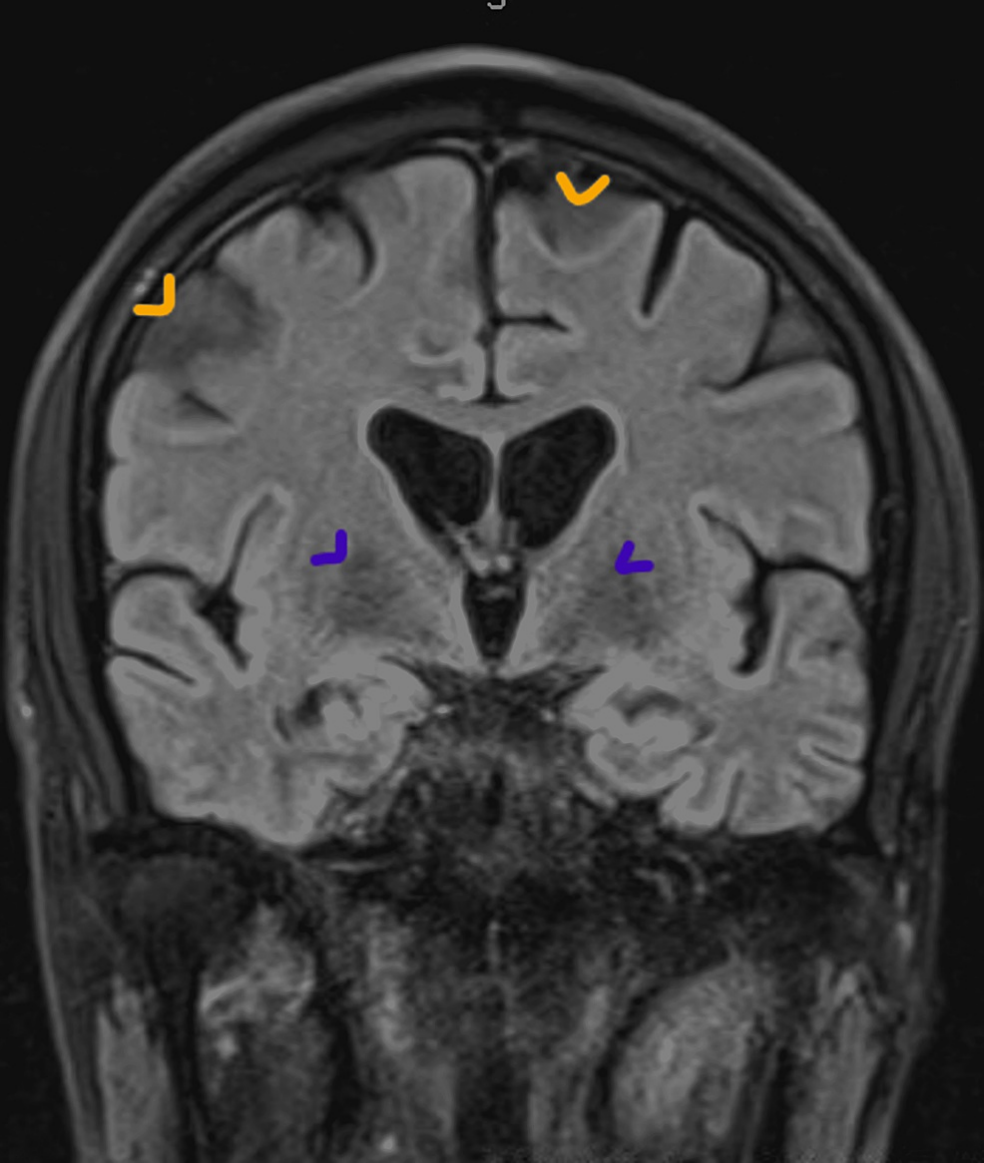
Patient's MRI. T2/FLAIR shows nonspecific hyperintensities in the periventricular (purple arrows) and subcortical (yellow arrows) regions, which is otherwise unremarkable. FLAIR: fluid-attenuated inversion recovery
